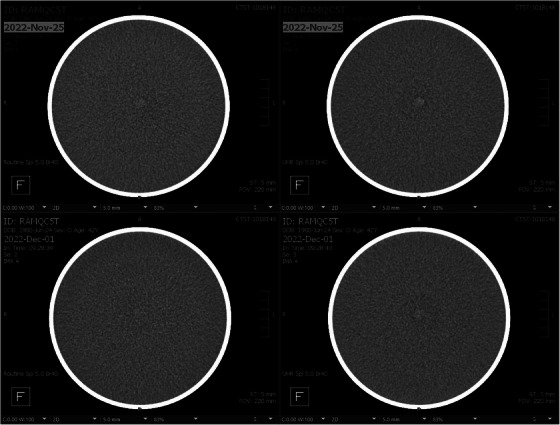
Daily CT QC images from the PCD‐CT system. Top row, initial data acquired in spiral mode with the standard (144 × 04 mm; left) and UHR (120 × 0.2 mm; right) detector configuration. Hyperdense artifact at the isocenter was noted, most prominent in the UHR mode, which contributed to the decision to replace detector modules during a scheduled system upgrade the following day. Bottom row, the same data acquired after the detector module replacement showed the artifact was strongly decreased. PCD,= photon‐counting‐detector; QC, quality control; UHR, ultra‐high‐resolution.
